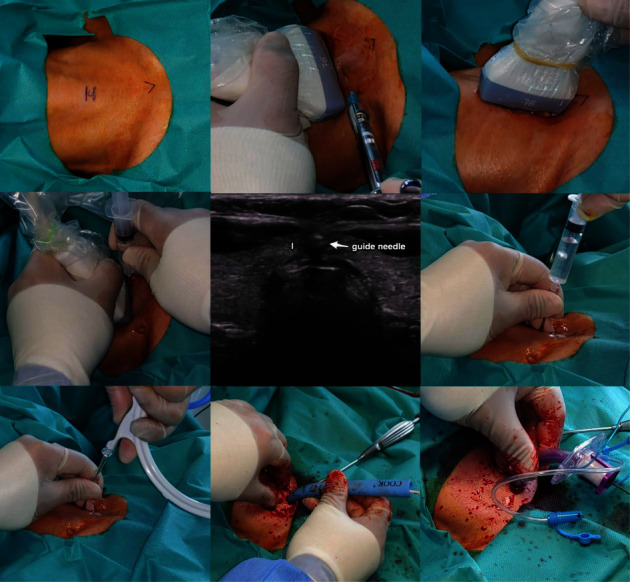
Real time ultrasound-guided percutaneous dilatational tracheostomy. After marking the cricoid cartilage and sternal notch, local anesthesia was injected superficial to the thyroid isthmus. Under sagittal conversion of the ultrasound probe, the ET tube was repositioned to an appropriate level to prevent tube puncture. The guide needle was inserted carefully to avoid damaging the cervical vasculature in the axial ultrasound view, followed by air regurgitation and insertion of the guidewire. The T-tube could be inserted along the guidewire following stepwise multiple dilatations using the Ciaglia Blue Rhino tracheostomy kit. I: thyroid isthmus, ET tube: endotracheal tube, T-tube: tracheostomy tube.
